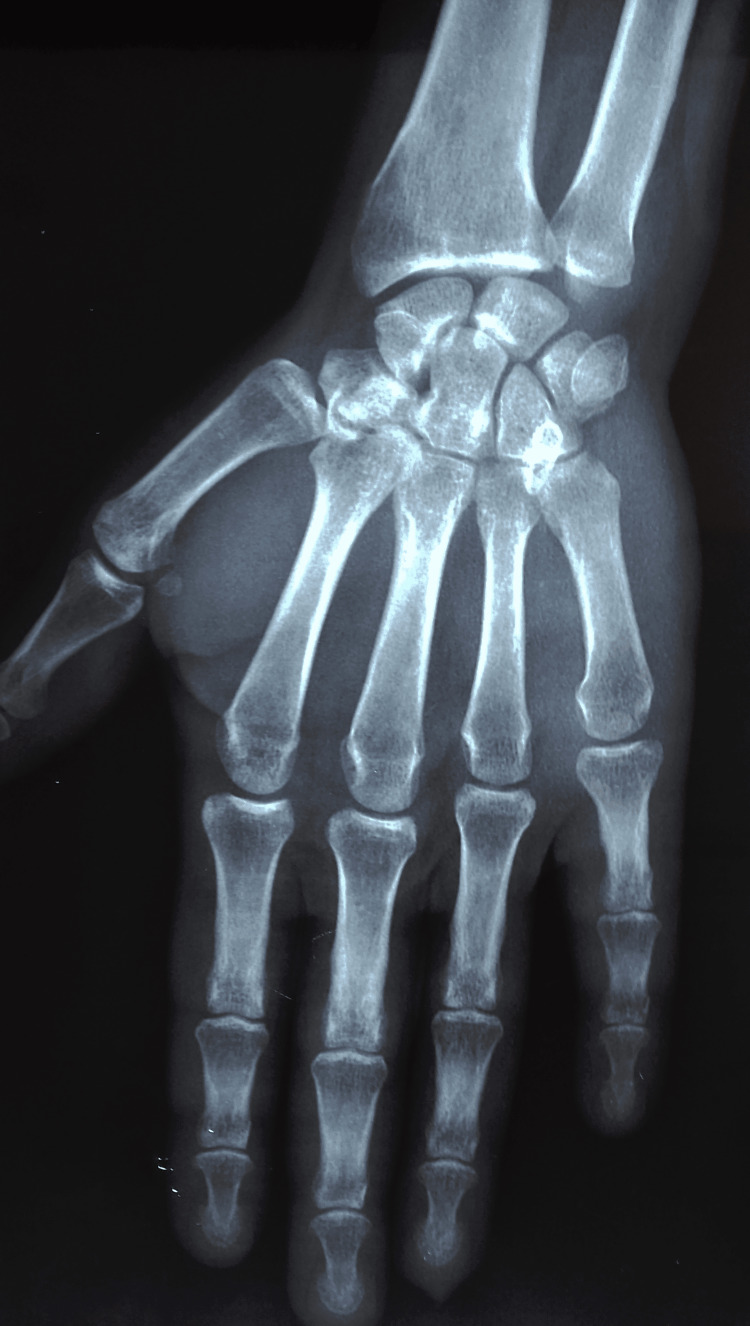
Radiograph showing anteroposterior view of the right wrist and hand
